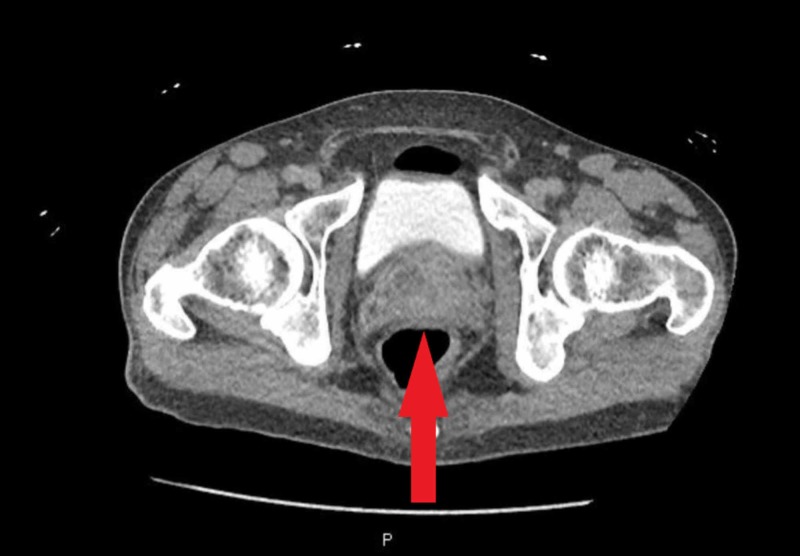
Prostate abscess extending into the seminal vesicles bilaterally seen on computed tomography scan with contrast
